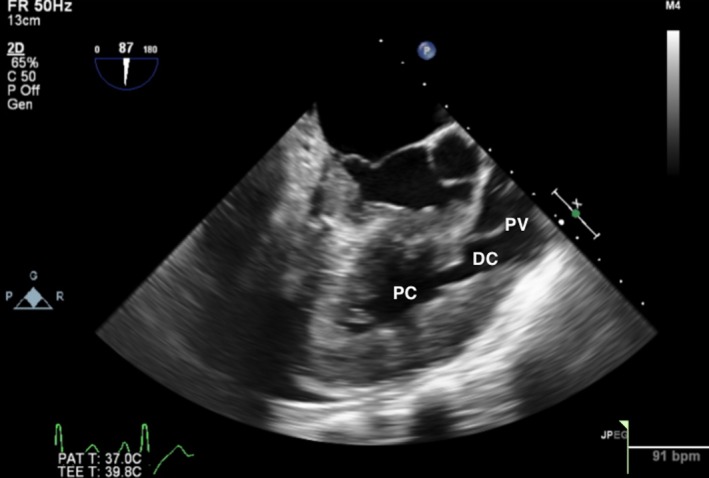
Transesophageal echocardiogram showing the right ventricle with a proximal chamber (PC) and distal chamber (DC) separated by a muscular band
